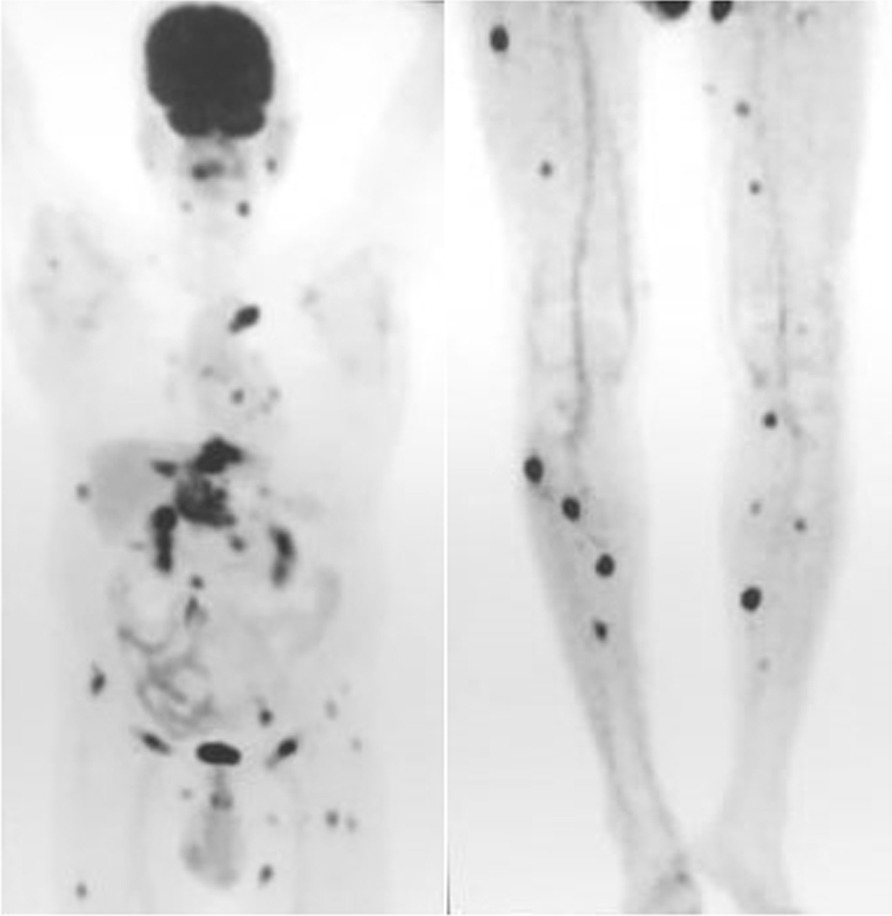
PET scan done after 6 months of bilateral axillary lymphadenectomy shows widespread metastasis. FDG avid lesions are visualized in the right sublingual, left pre-auricular, bilateral paraspinal lymph nodes, left internal mammary, bilateral hilar, subcarinal lymph nodes and left pleura and lower lobe. Metastasis to left lobe of liver, anterior abdominal wall at the previous operative site, omentum, peripancreatic, left external iliac, bilateral inguinal lymph nodes, bilateral paraspinal muscles, right intercostal muscles, bilateral gluteal muscles and multiple lower limb muscles is visible
